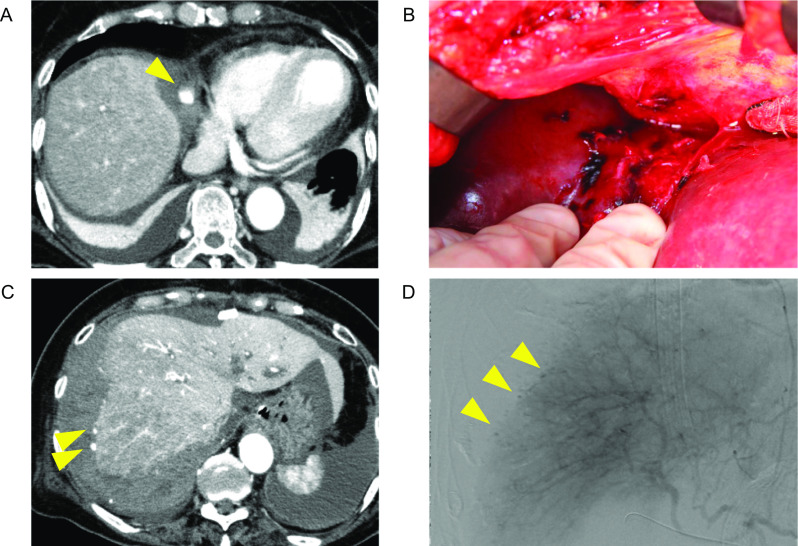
Findings of Case 1. A CE-CT (arterial phase) on POD2. The yellow arrowhead shows the extravasation of contrast medium from the hepatic falciform ligament artery. B Intraoperative findings of hemostatic surgery on POD2. C CE-CT (arterial phase) on POD 8. Yellow arrowheads show the extravasation of contrast medium from the liver surface. D The findings of angiography of the hepatic artery on POD8. Yellow arrowheads show the extravasation of contrast medium from the liver surface. CE-CT: contrast-enhanced computed tomography; POD: postoperative day
